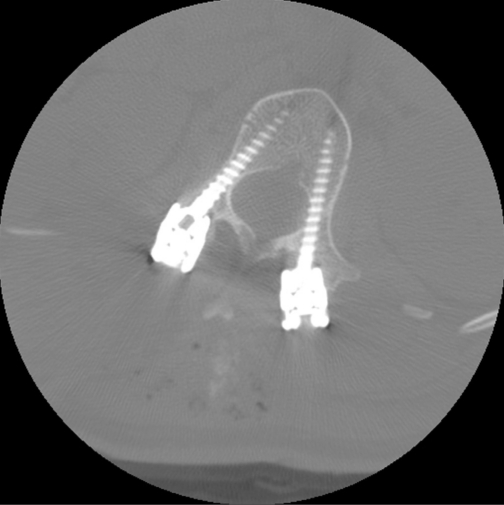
Axial postoperative CT showing medial breach of L1 pedicle. Note the significant rotational component to the patient’s scoliosis
